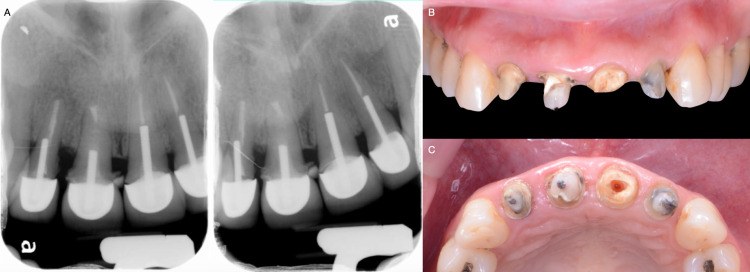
A) Initial radiographs and B) Clinical evaluation after crown removal.
